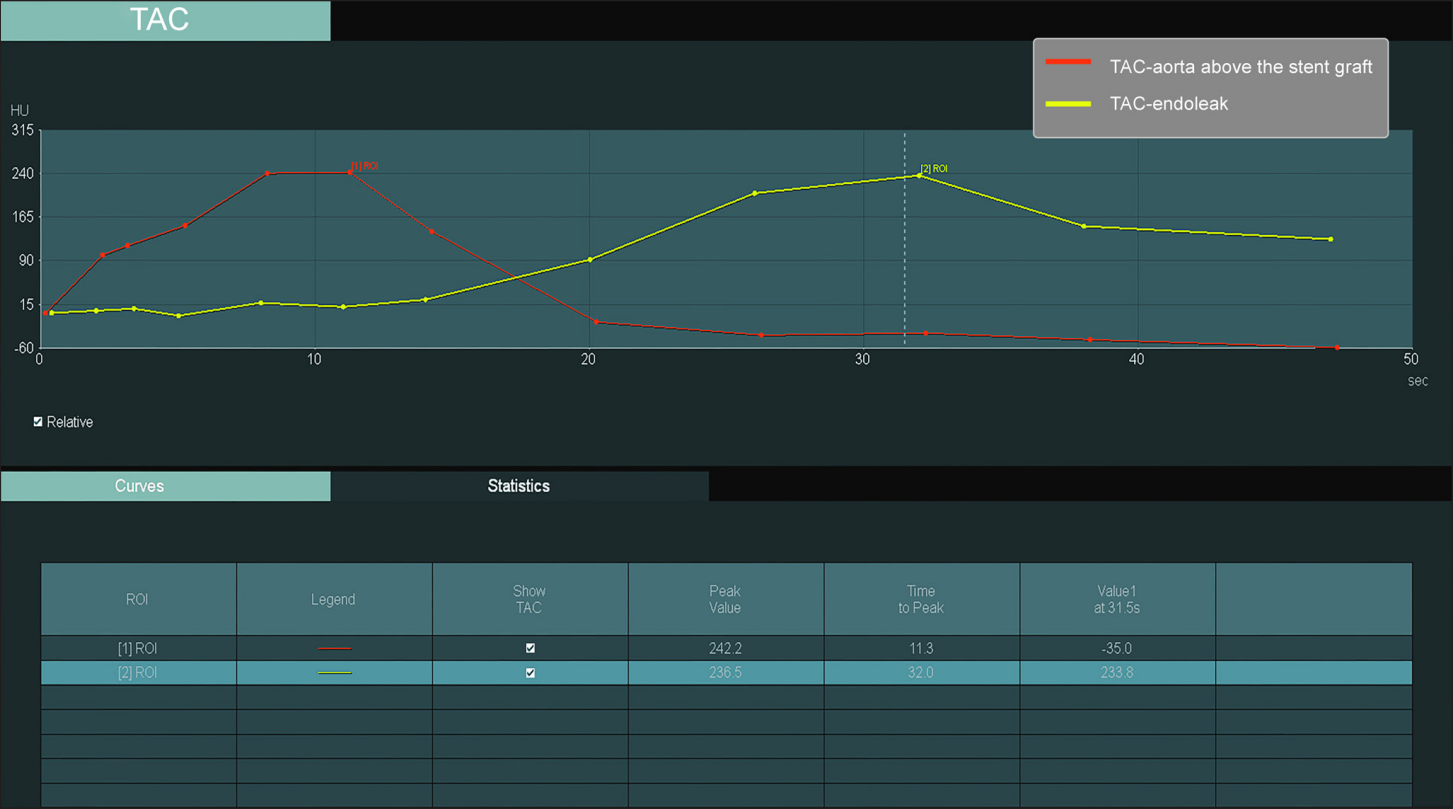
Time attenuation curve (TAC) of the aorta and the site of endoleak pertaining to Figure 2 dynamic CTA (d-CTA). The TAC of the d-CTA shows a significant delay (20 seconds) in the peak of the Hounsfield unit between the region of interest (ROI) placed in the aorta (red ° sign, ROI-1) and ROI placed at the site of the endoleak (yellow # sign, ROI-2), suggesting the sac filling from the inferior mesenteric artery toward the aneurysm sac rather than direct filling from the aorta.
